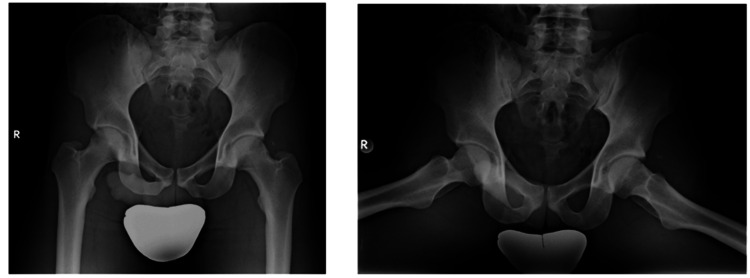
Final follow-up radiographs at five years showing no avascular necrosis and joint dysfunction.
